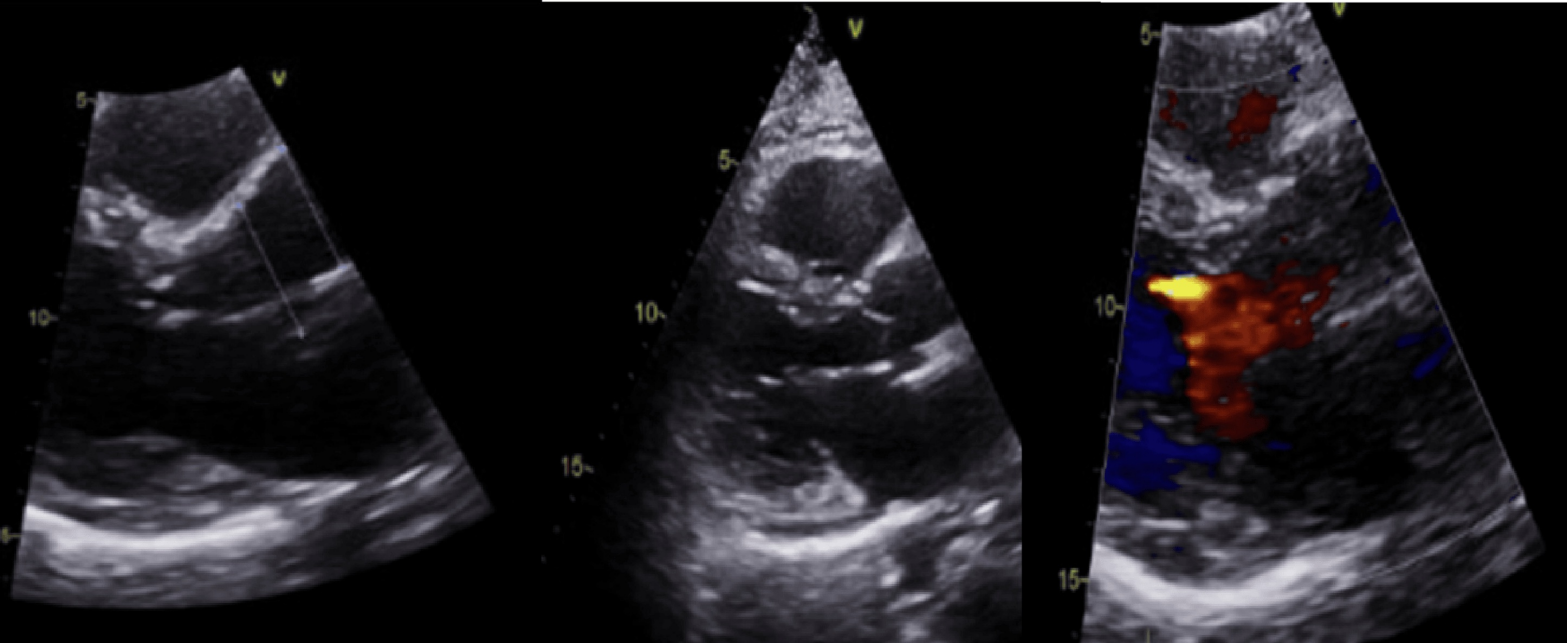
Transthoracic echocardiogram images Normal left ventricular size and systolic function, normal right ventricular function, normal sizes of both left and right atria, and no clinically significant valvular abnormalities or pericardial effusion.
